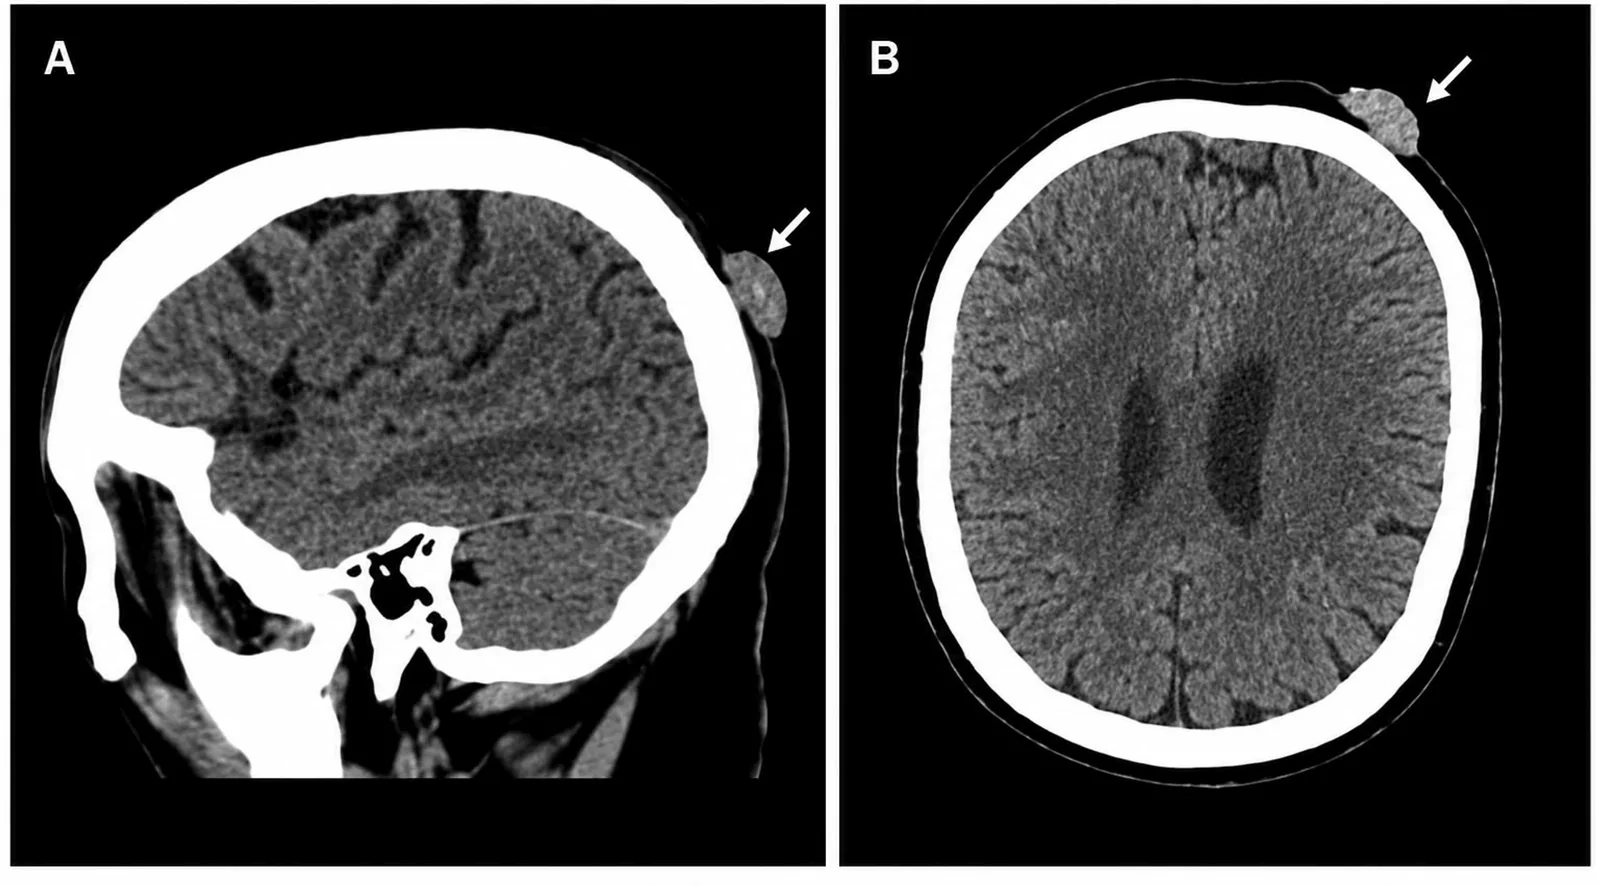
Preoperative computed tomography (CT) of the head demonstrating a scalp lesion without underlying calvarial or intracranial invasion. Sagittal (A) and axial (B) CT images demonstrate a left occipital scalp mass without evidence of calvarial erosion, intracranial extension, or cervical lymphadenopathy.
